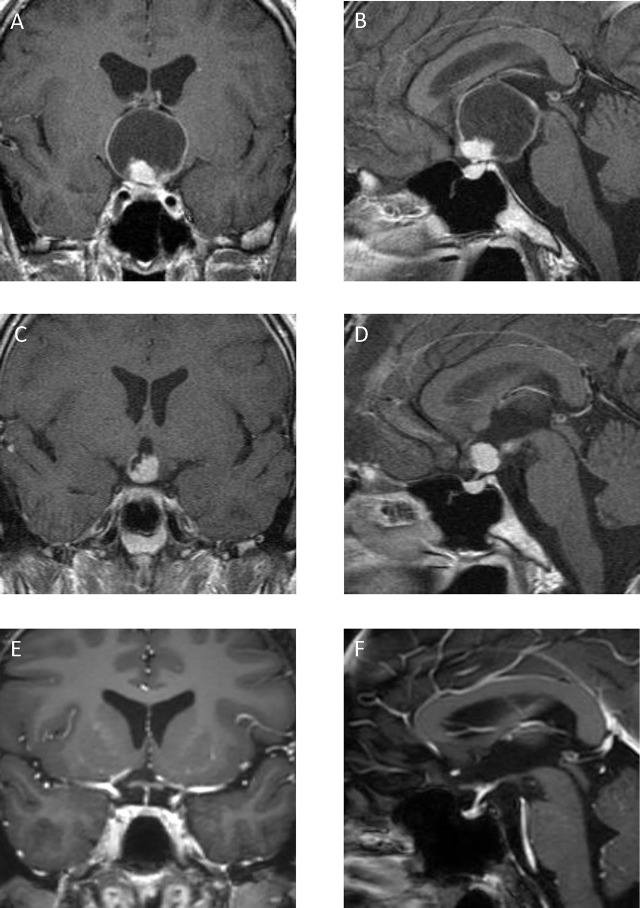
Case 2: An 18-year-old man. Preoperative coronal (A) and sagittal (B) enhanced T1-weighted magnetic resonance imaging (MRI) showed a mainly cystic tumor, which extended to the third ventricle. Postoperative enhanced T1-weighted MRI showing a residual tumor in the third ventricle (C, D). There was no tumor recurrence after performing a combination of conventional local irradiation and Gamma Knife radiosurgery (E, F).
